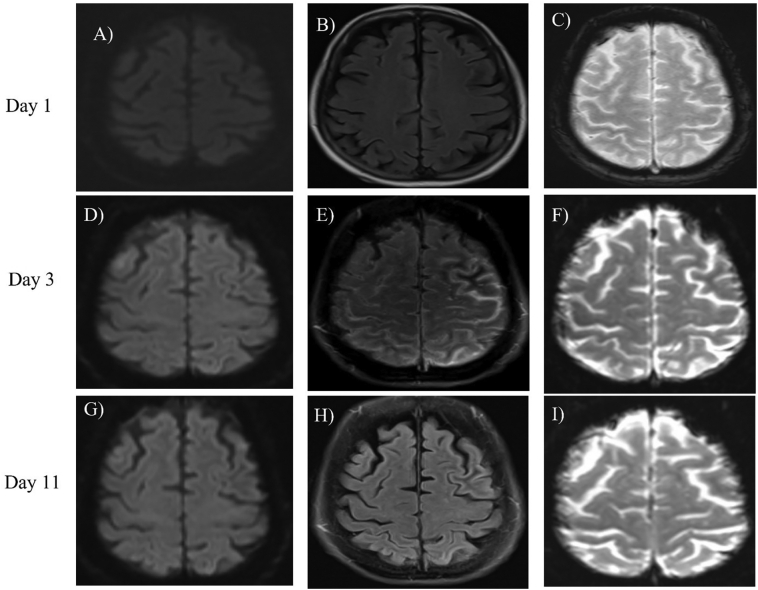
A–C: Axial images from brain MRI with three different sequences (DWI, FLAIR and GRE, respectively) at the time of presentation showing no abnormalities. D-F: Axial MRI images (in above sequence): Subarachnoid hyperintensity on FLAIR sequence (E) over the left parietal and frontal convexity with no corresponding changes on DWI (D) or GRE (F). G-I: Axial MRI images (in above sequence), showing complete resolution of the subarachnoid FLAIR hyperintensity (H) along the left parietal and frontal convexity with no abnormalities on DWI (G) or GRE (I).
